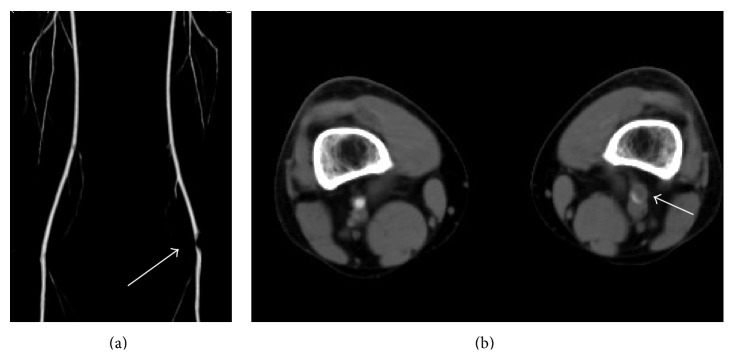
Computed tomography shows (a) the occlusion of the left popliteal artery (white arrow) and (b) a cystic mass compressing the popliteal artery (white arrow).
